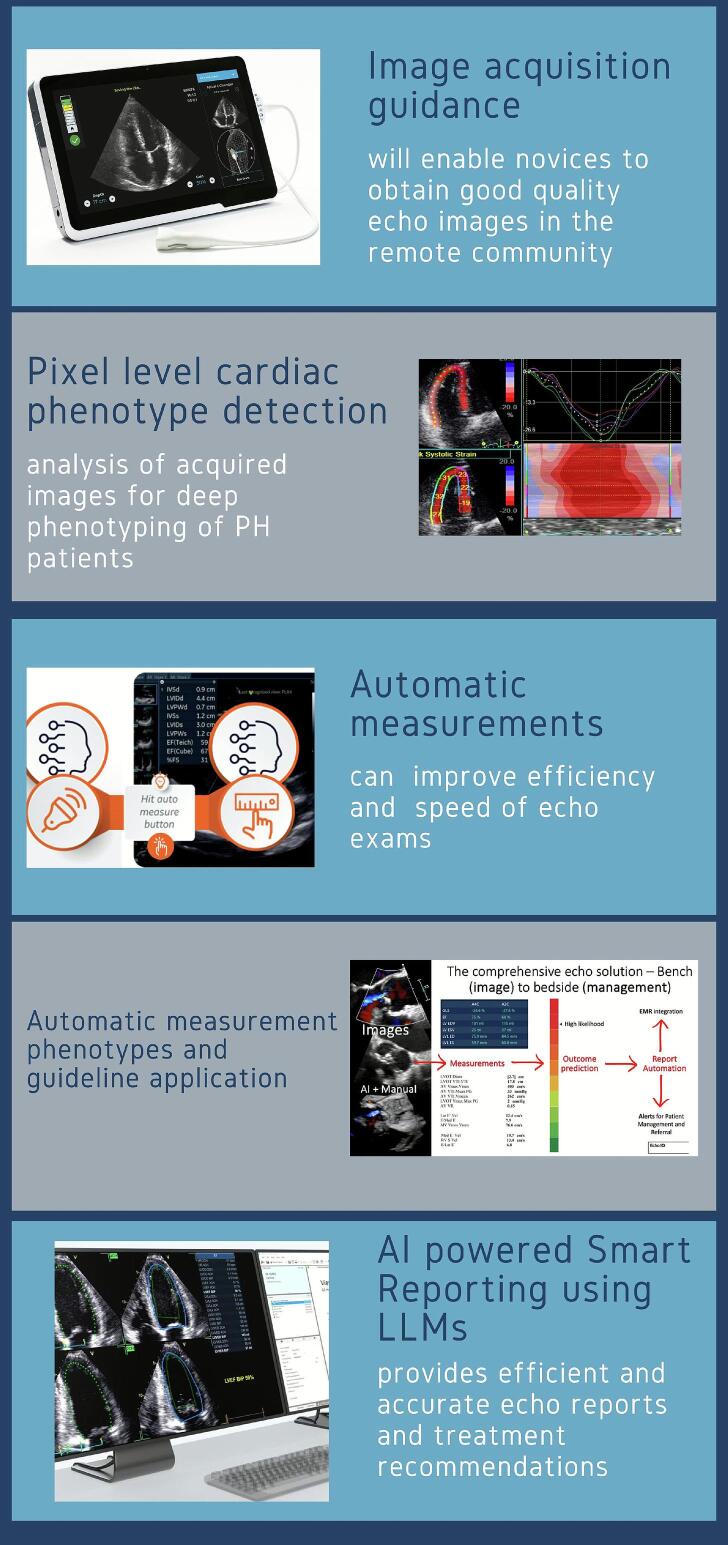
Artificial intelligence (AI) enhanced echocardiography for optimizing diagnosis of PH in isolated populations. Improved triaging of patients with the use of AI will help to inform the most appropriate imaging test for the patient. If echocardiography is appropriate, improved image quality may be obtained using AI guidance, followed by AI analysis of images for automated measurements or phenotype detection. Measurement data obtained may then be subject to further AI for application of clinical practice guidelines and risk models, and large language models (LLMs) for automation of echocardiographic reporting in preparation for review by an expert physician. AI synthesis of echocardiographic and clinical information may assist in decisions to further investigate at-risk patients with possible PH.
